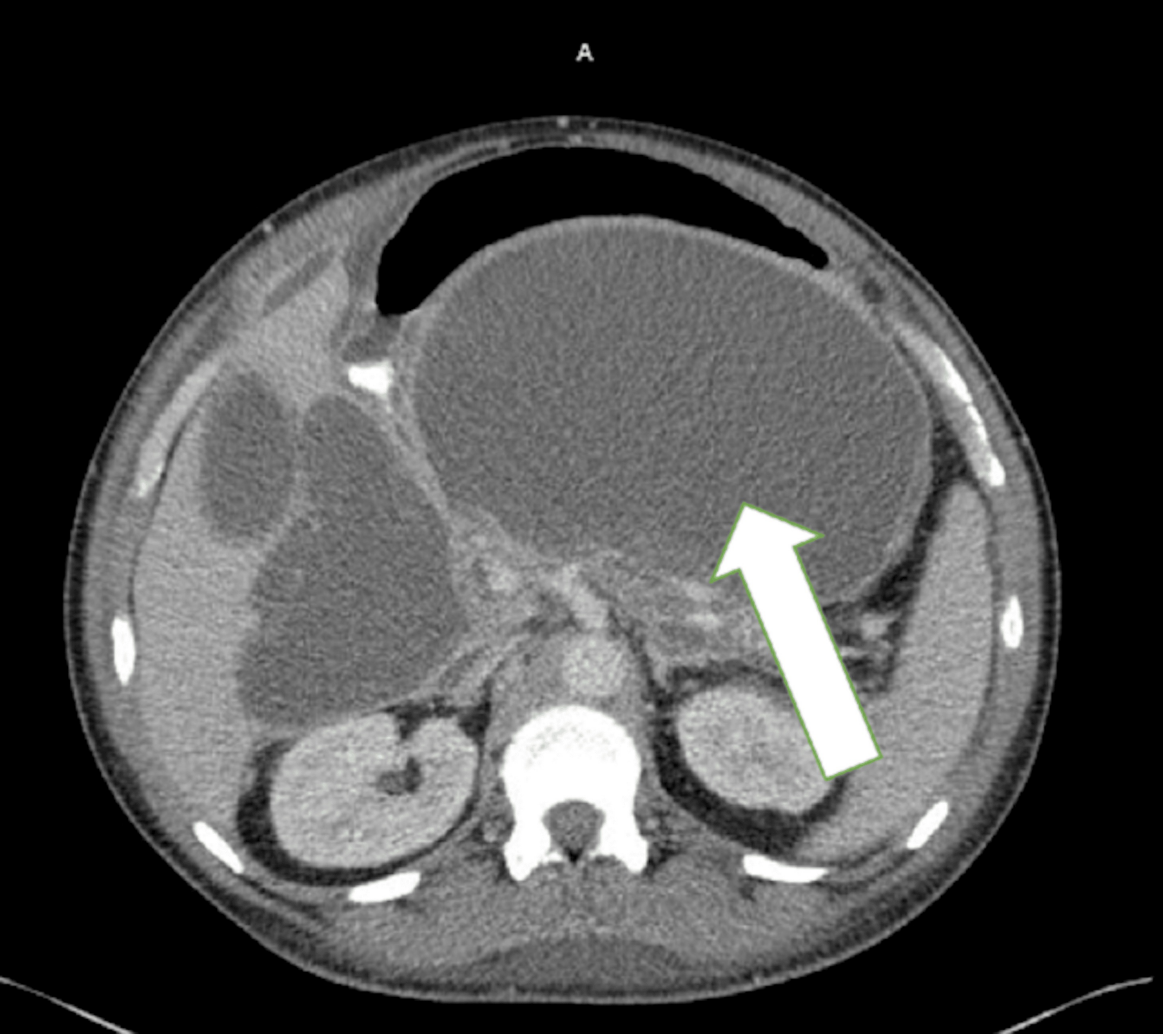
Axial computed tomography image (contrast-enhanced) A well-encapsulated cystic lesion (white arrow) is observed in the pancreatic region, compressing surrounding structures without significant internal septations.
